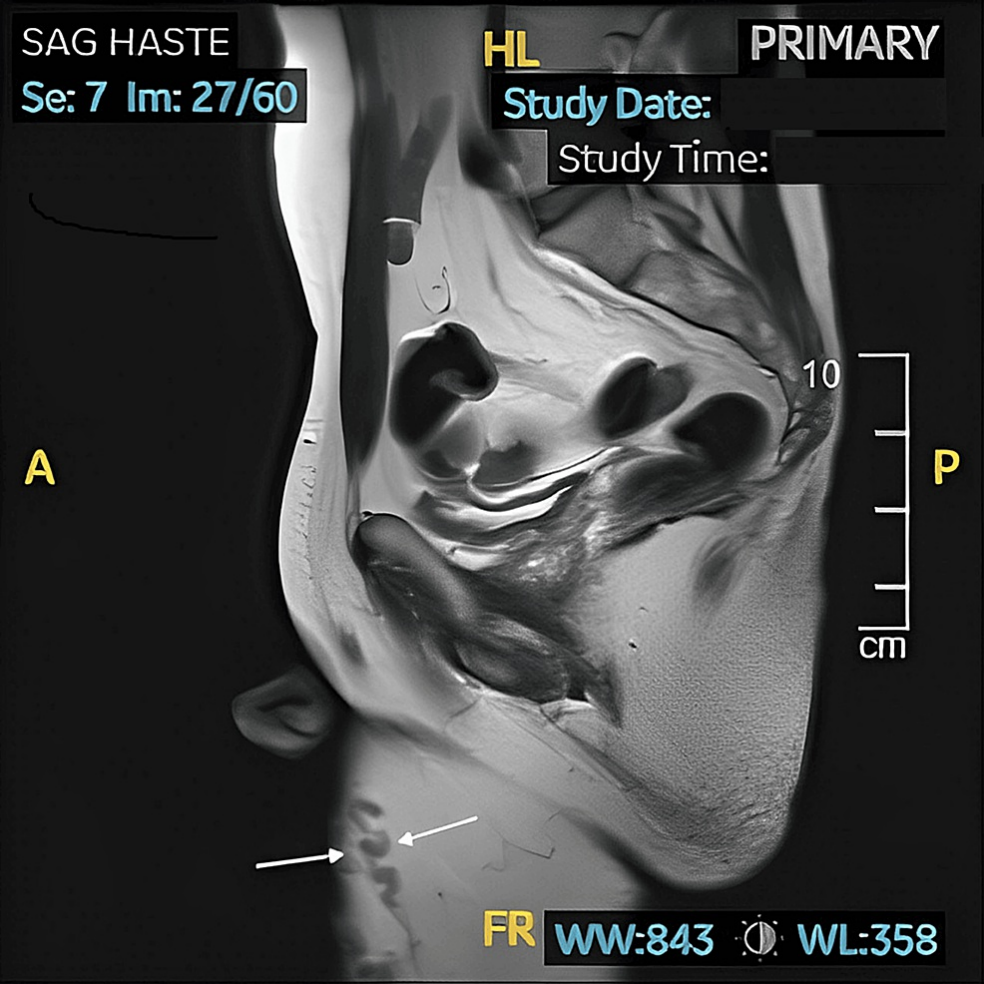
MRI of the pelvis showing venous varicosities seen in the anterior right thigh extending into the pelvis
